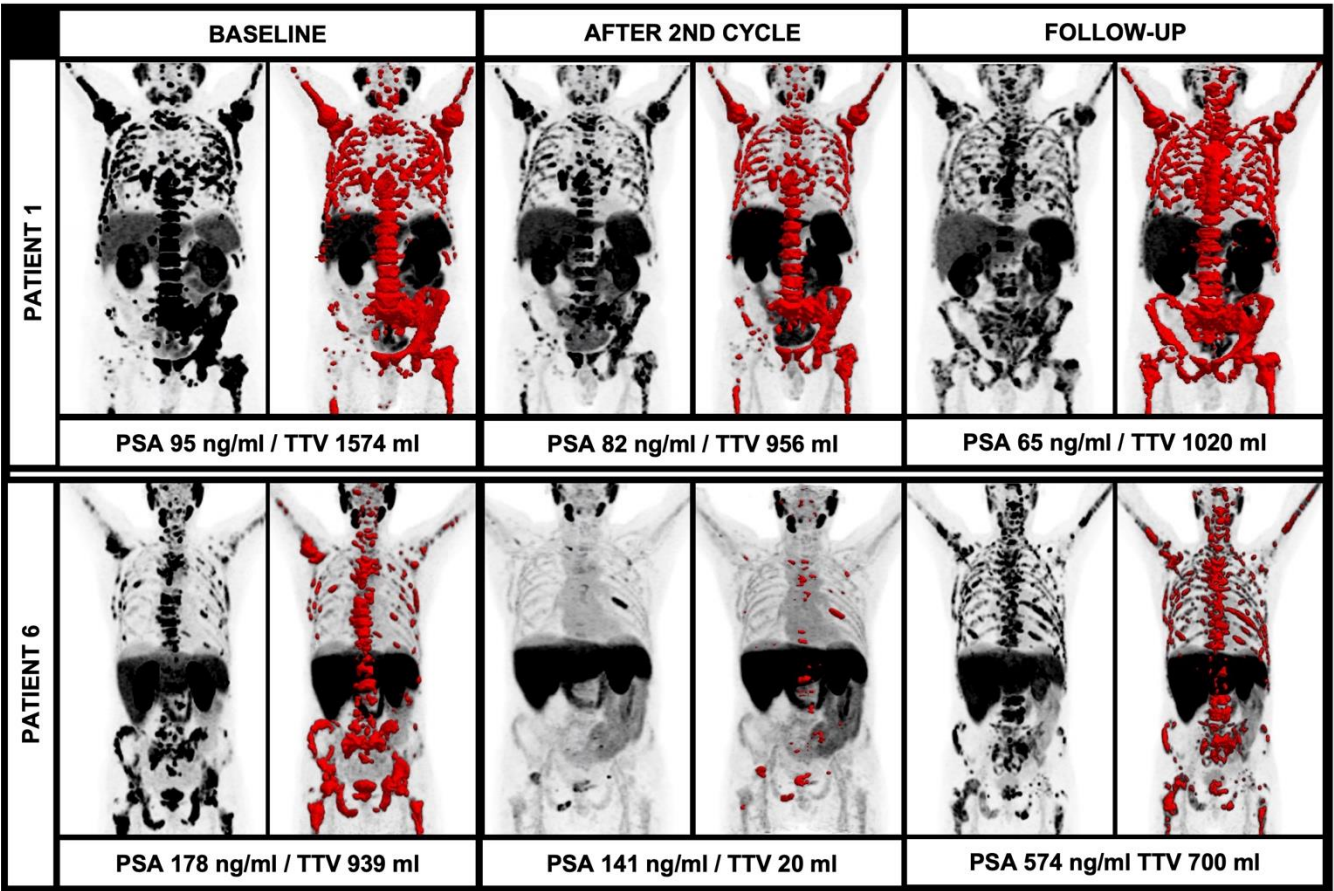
Diverging course of TTV with newly occurring lesions during alpha therapy. While patient 1 had a stable TTV and decreasing PSA during therapy despite new PSMA-avid lesions, patient 6 had new PSMA-avid lesions with an extensive increase of TTV and PSA.
